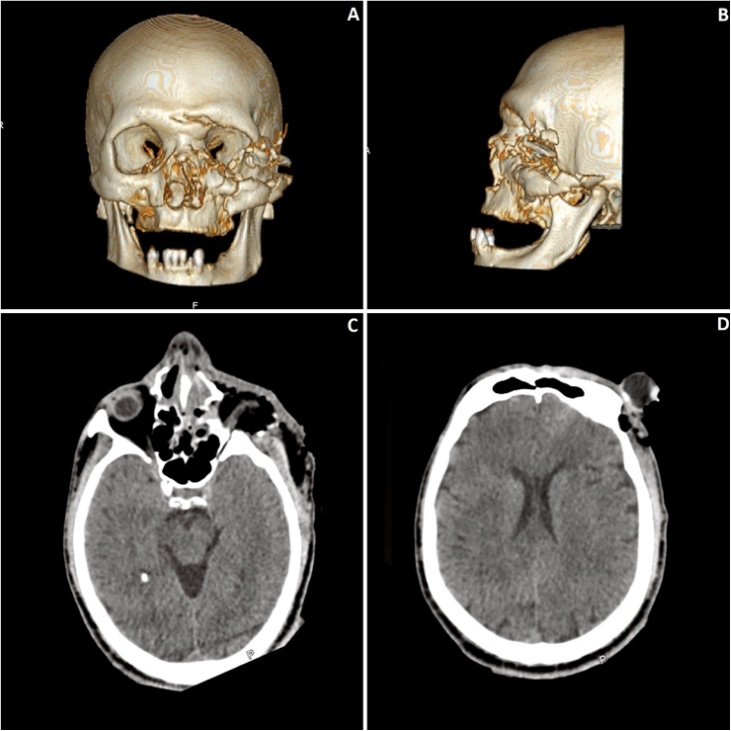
Pre-operative CT scan. Frontal (A) and lateral view (B) of a 3D CT reconstruction showing a panfacial fracture with comminution of the left orbitozygomatic complex. Axial cuts showing a sectioned optic nerve (C) and a the displaced left globe (D).
